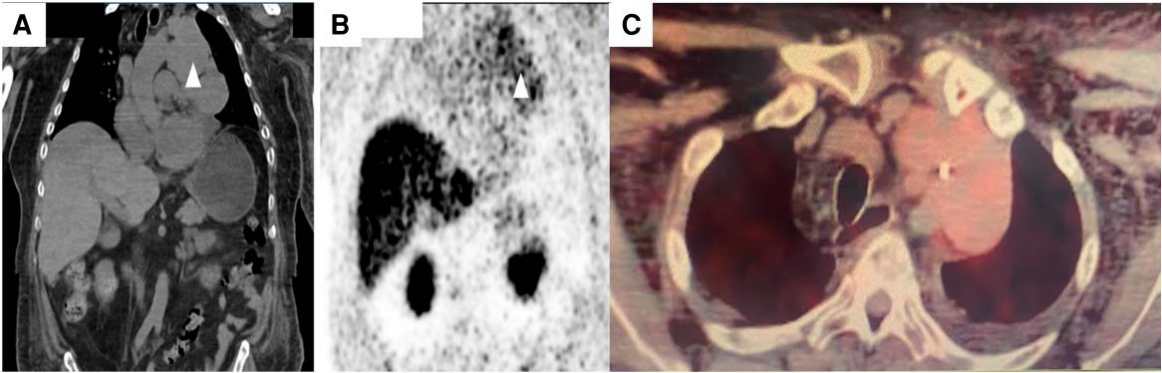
(A) Contrast-enhanced CT image showing a 13.5 × 6.4 cm mediastinal tumor (white arrow). (B) Scintigraphy demonstrating physiologic uptake of 18F-NOTA-octreotide in the liver and adrenal glands, and pathologic uptake in the mediastinal tumor (white arrow). (C) Axial fused PET/CT with 18F-NOTA-octreotide showing heterogeneous uptake in the mediastinal tumor with an SUVmax of 3.1.
